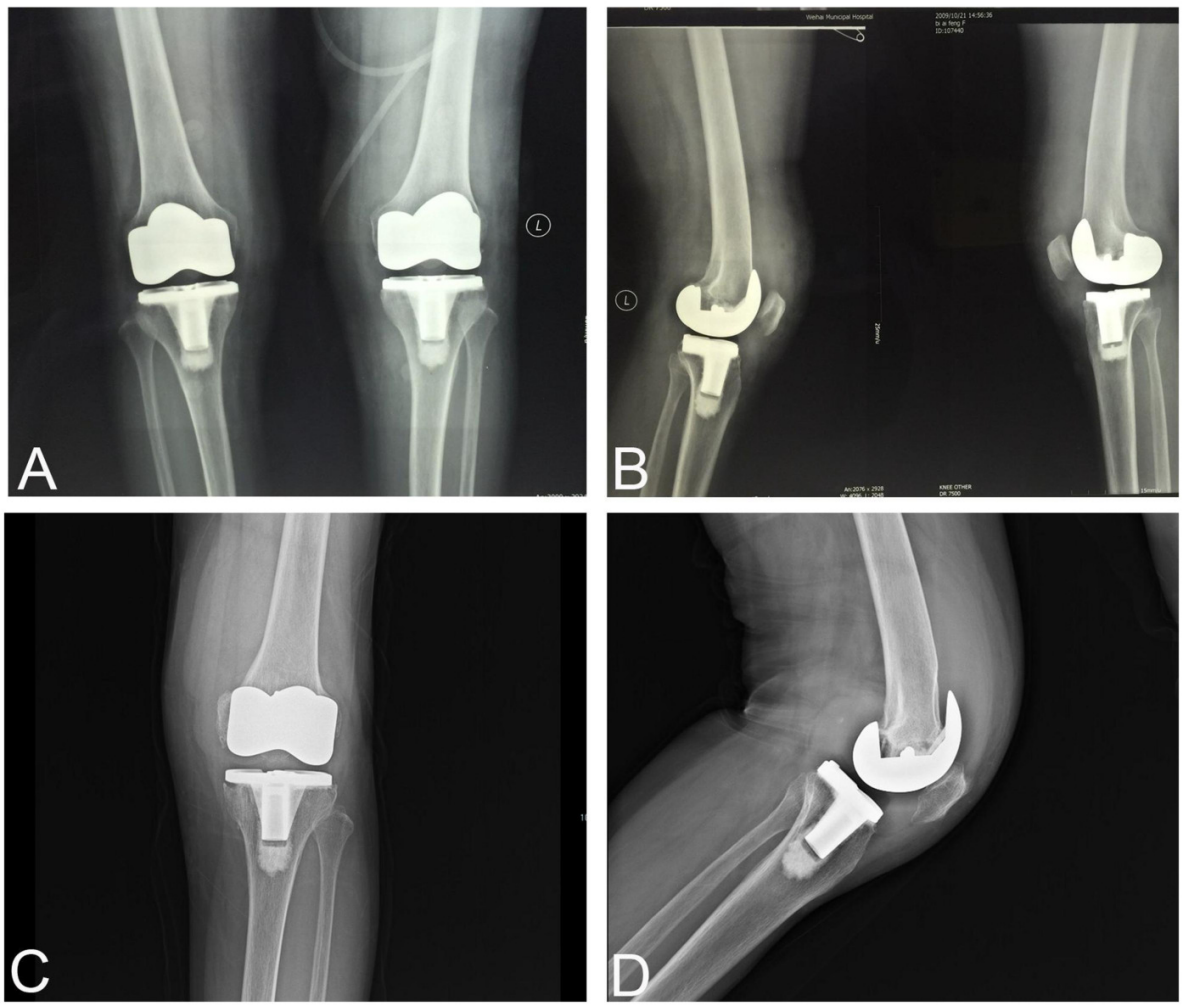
(A) Anteroposterior radiograph after the initial total knee arthroplasty in 2009. (B) Lateral radiograph after the initial surgery in 2009. (C) Anteroposterior radiograph after left knee symptom aggravation in 2016. (D) Lateral radiograph after left knee symptom aggravation in 2016.
